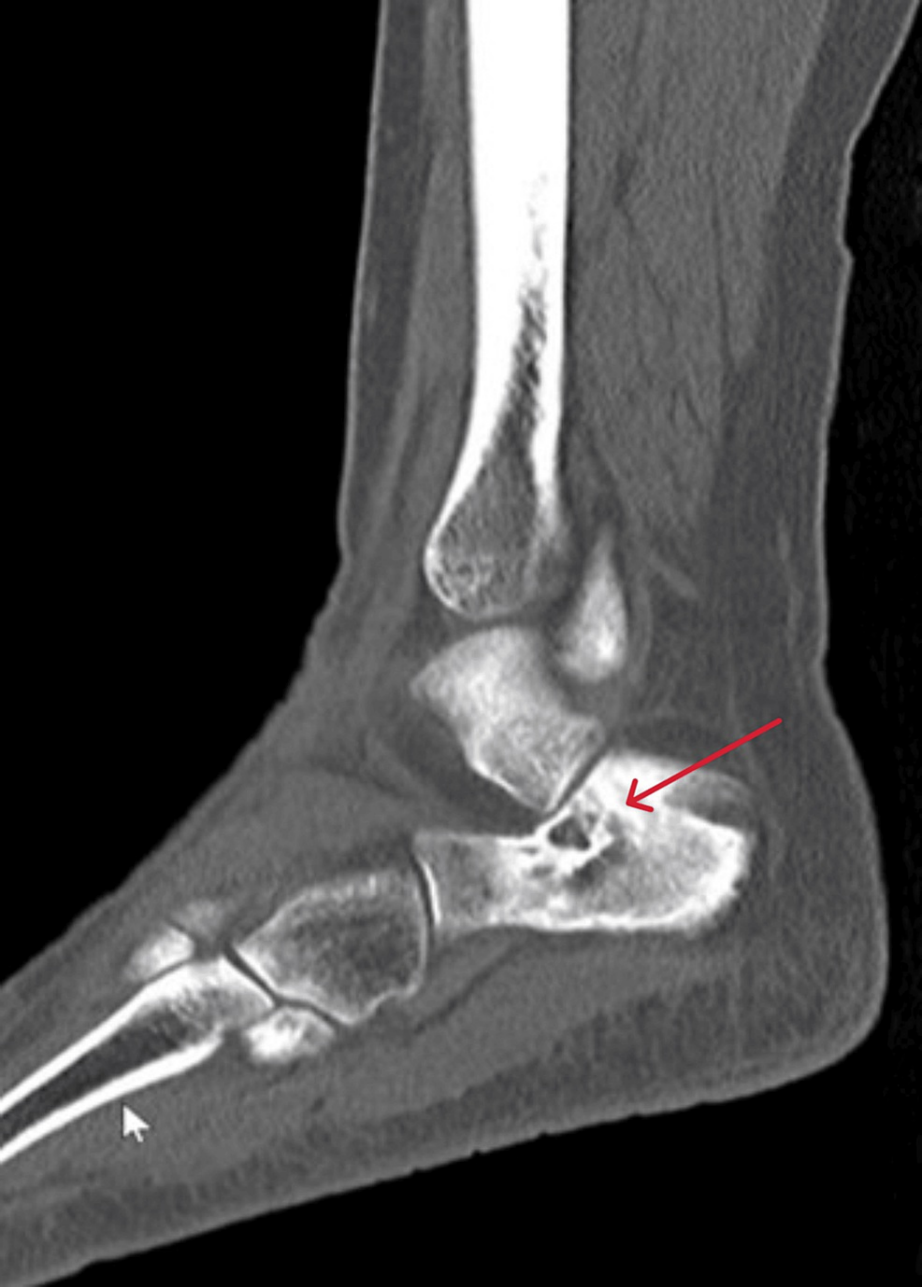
Sagittal view CT scan of the right ankle at one-and-a-half-year follow-up CT: computed tomography
